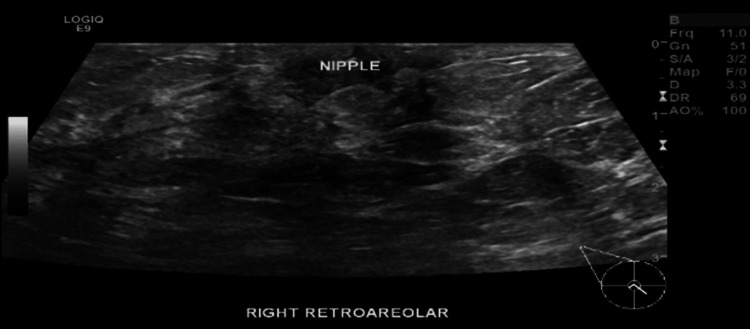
Right breast ultrasound showed complete resolving of the breast abscess
